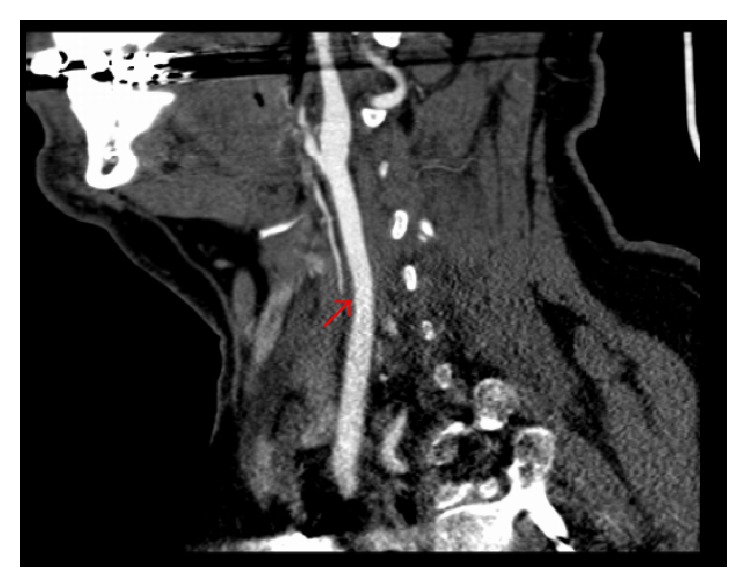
Sagittal CT angiogram of the neck (3 months later) showing very minimal mural thrombus remaining.
